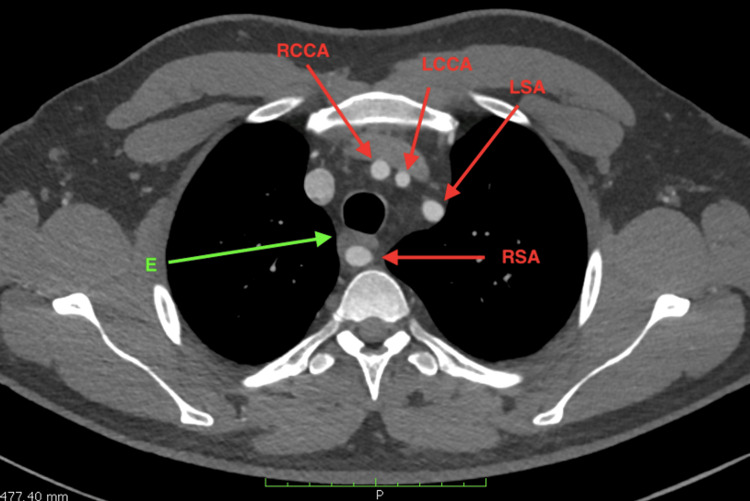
CT angiography: horizontal section at the level of the third thoracic vertebra. The RCCA, LCCA, and LSA are identified. The RSA is seen ventral to the third vertebral body, causing significant compression of the esophagus. CT: computed tomography; RCCA, right common carotid artery; LCCA, left common carotid artery; E: esophagus; LSA: left subclavian artery; RSA: right subclavian artery.
